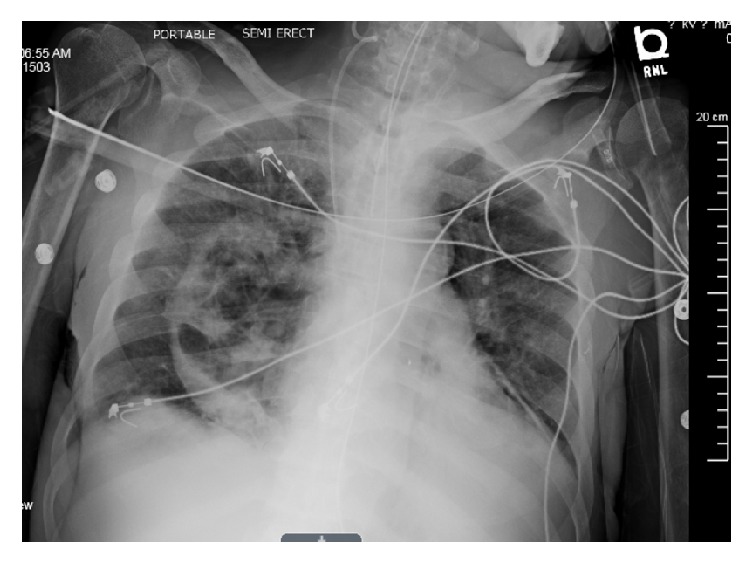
Chest X-ray showing a new patchy right lower lobe opacity consistent with pneumonia and persistent pneumopericardium (day 17).
